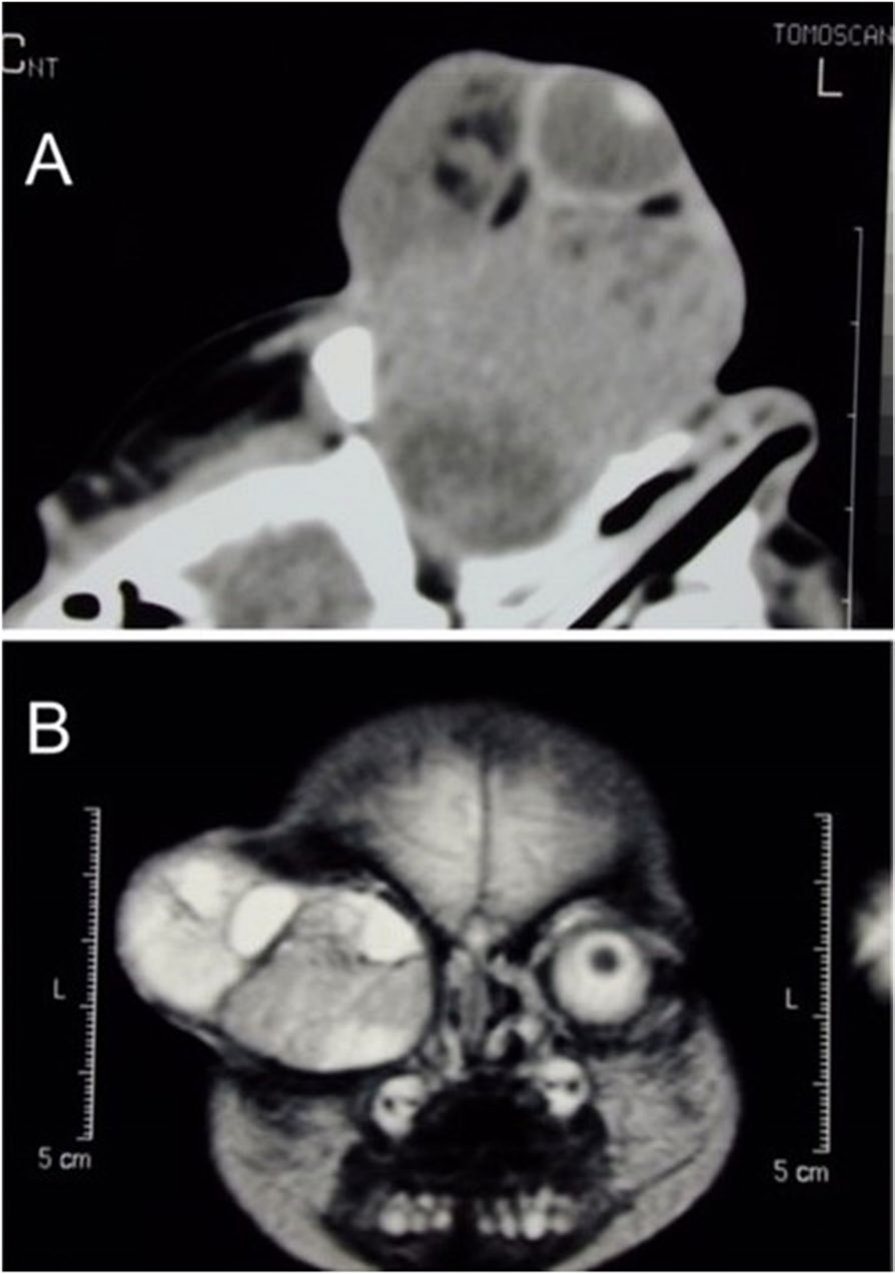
a CT of the orbits shows a large heterogeneous mass, filling the entire orbit. b T2-weighted MRI shows the tumor to be hyperintense to fat and extraocular muscles
